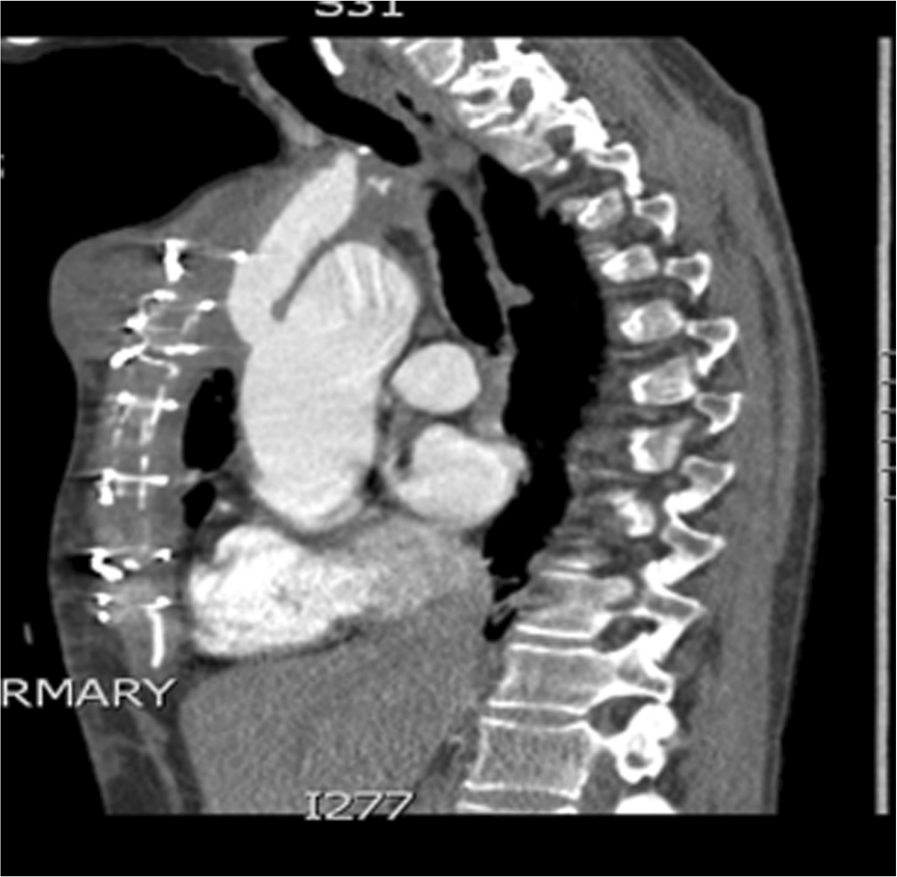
CT scan showing subcutaneous collection overlying manubrium, the top sternal wire with its posterior tip precariously close to the innominate graft, although there is no suggestion of the subcutaneous collection communicating with the innominate graft.
